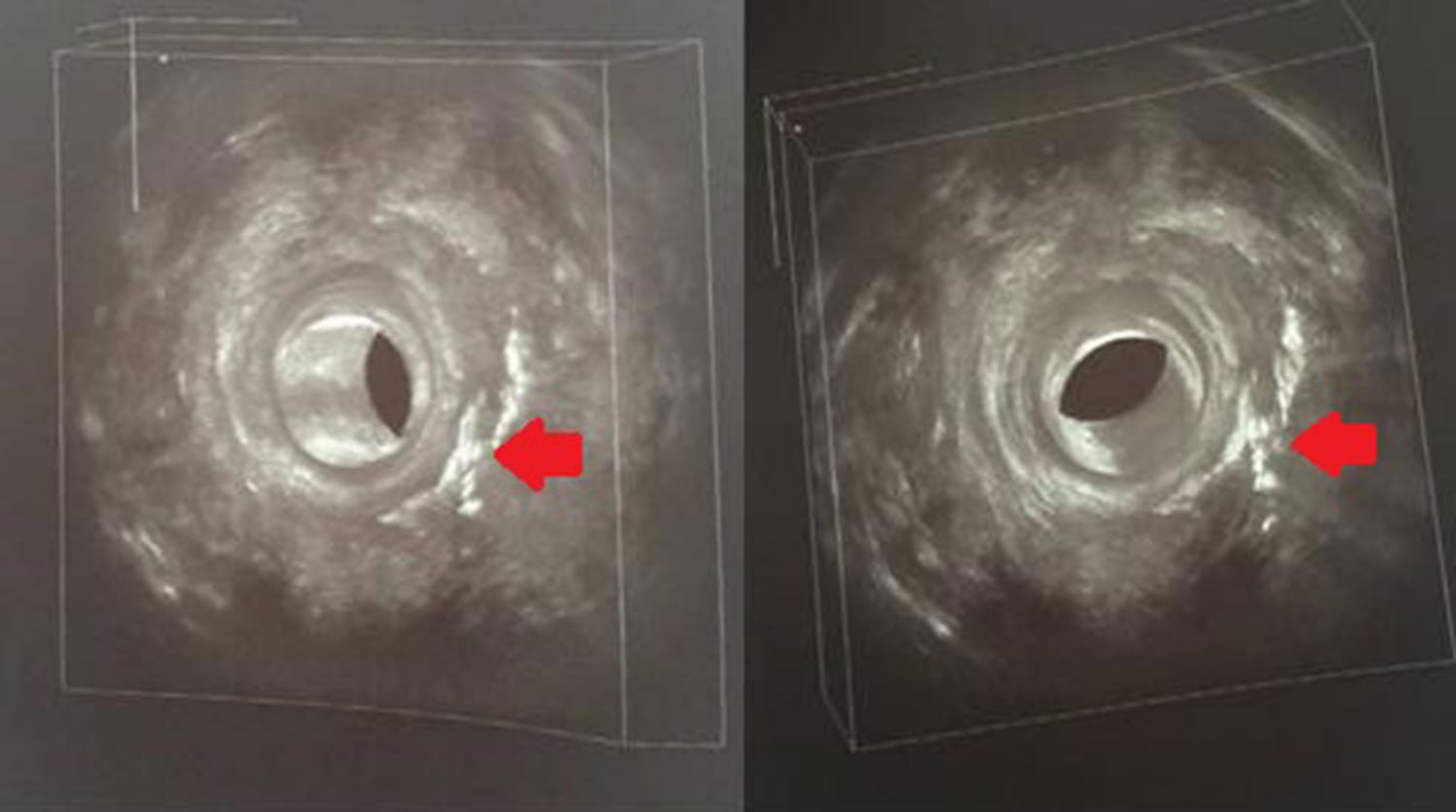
Preoperative transanal ultrasound showing the fistula (red arrow)
